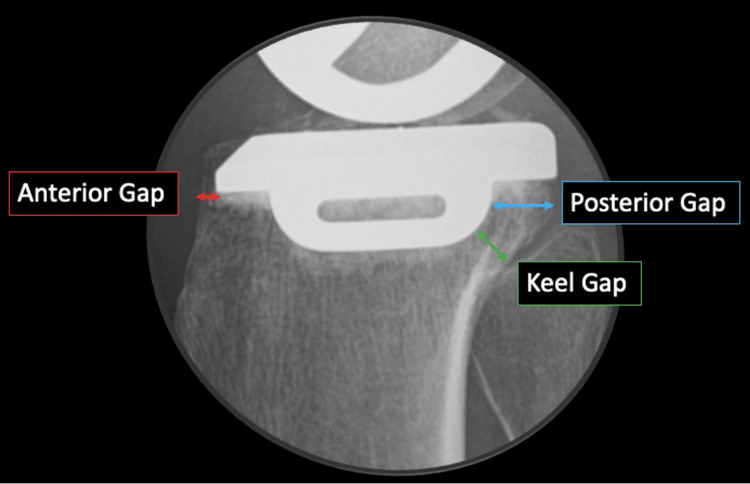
Postoperative radiographs (lateral view) of the patients Anterior gap: distance from the anterior tibial cortical bone to the tibial component. Keel gap: the shortest distance from the posterior margin of the keel to the posterior cortical bone. Posterior gap: horizontal distance from the posterior margin of the keel to the posterior cortical bone.
